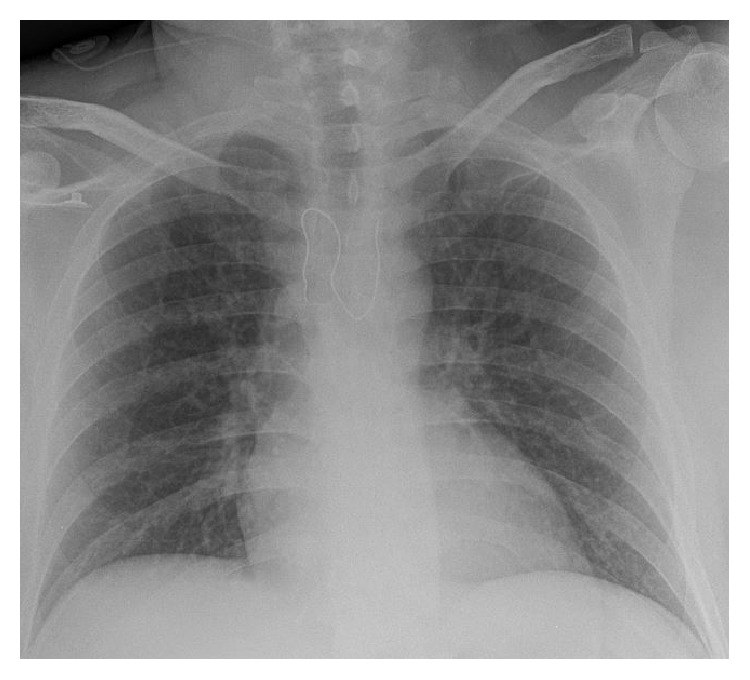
Chest X-ray showing a curvilinear metallic object overlying the upper mediastinum.
